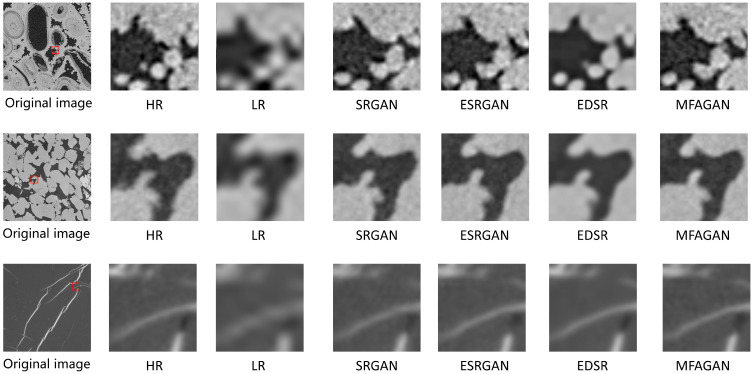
Comparison of super-resolved core CT images reconstructed by different methods. The location of the HR image in the original image is indicated by the red box.
